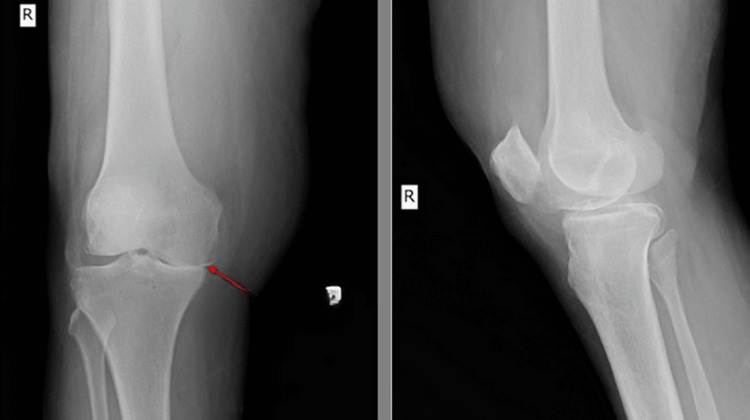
Right knee radiograph AP and lateral projections showing a reduction in joint space (medial>lateral) with osteophytes (red arrow) suggestive of osteoarthritic changes. AP: anteroposterior
